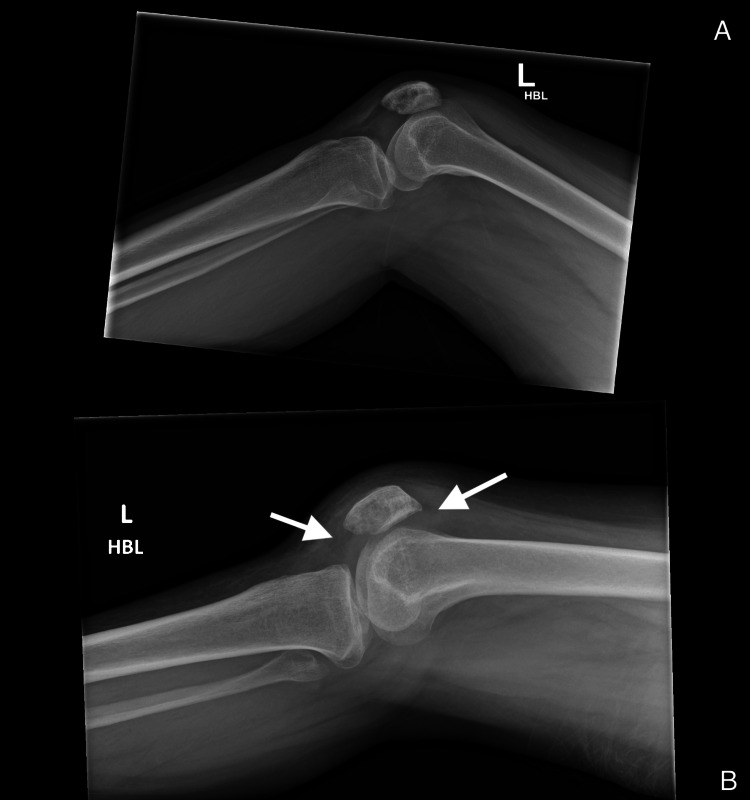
Radiographs of her left knee. (A) Five months prior to her initial presentation. (B) Five months post-fall and upon initial presentation to the orthopaedic team (A) No evidence for any cortical disruption or a joint effusion suggestive of fracture or soft-tissue injury. (B) Five months after her initial fall. The lateral knee radiograph shows a small effusion, which is highlighted by the arrows
